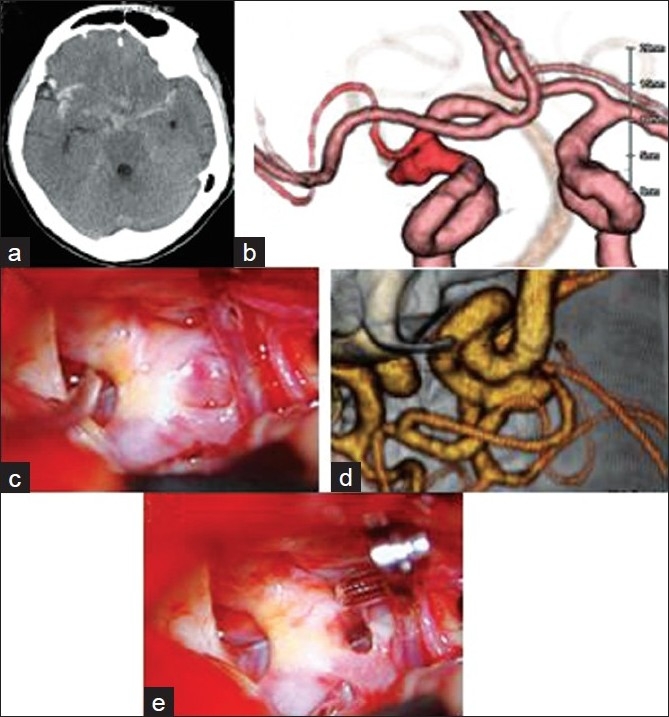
(a) CT scan on admission revealed a diffuse SAH with laterality on the right Sylvian fissure. (b and d) A 3D-CTA showed an ICA aneurysm located at the origin of hypoplastic dup-MCA associated with an acc-MCA arising from the anterior cerebral artery to share in supplying the right MCA territory. (c) The aneurysm measured 6 mm in size and was directed laterally. An intraoperative photograph showing the dup-MCA, the acc-MCA, and the A1 segment of the anterior cerebral artery. After the dissection of the carotid cistern, we traced the right ICA, and observed that the dup-MCA arose from the proximal ICA with an aneurysm arising from the trunk of these vessels. (e) The dup-MCA projected toward the Sylvian fissure. With the further retraction of the frontal lobe, aplasia of the main MCA was confirmed. The neck of the aneurysm was thereafter dissected and clipped successfully
